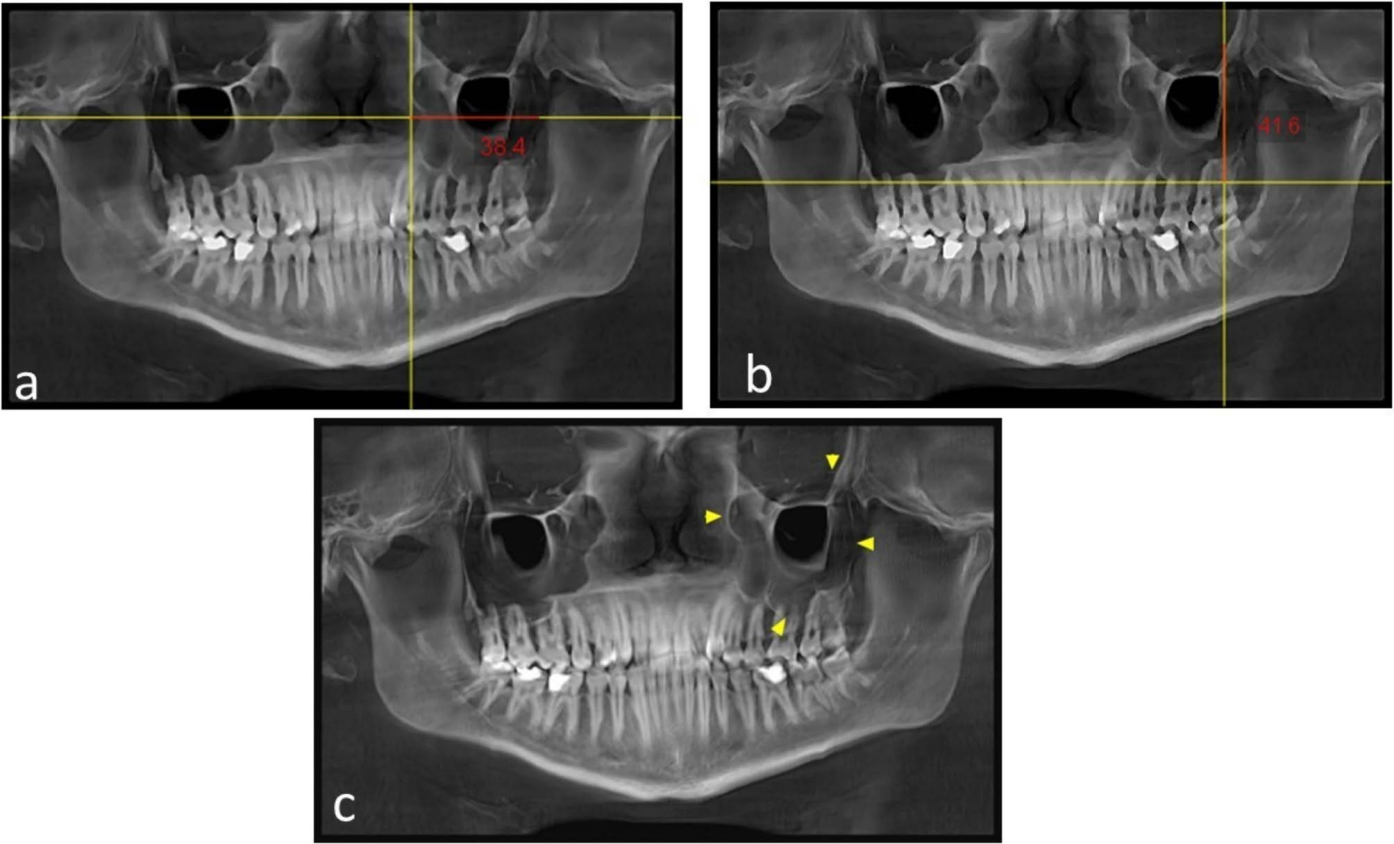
a CBCT reformatted panoramic image showing the Max. maxillary sinus width (red line). b CBCT reformatted panoramic image showing the Max. maxillary sinus height (red line)Max. maxillary sinus height in mm (Fig. 4b, c).
